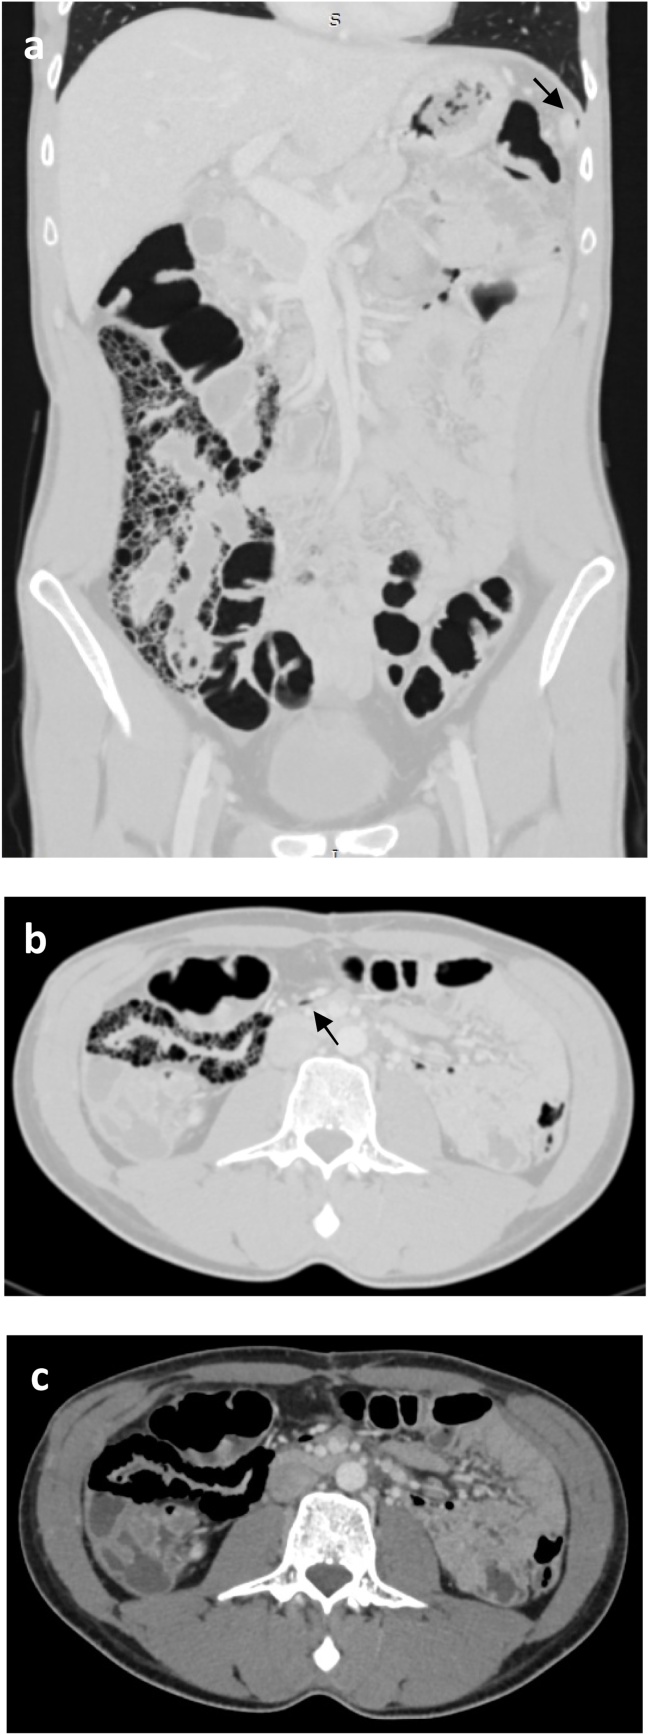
Portal enhanced CT-scan of the abdomen and pelvis: Coronal and axial views in lung window setting (a, b) and axial view in soft tissus window setting (c) showing gas in multiple small cysts within the wall of the terminal ileum. Presence of pneumoperitoneum under the left hemi diaphragm and within the mesenter (arrowheads).
